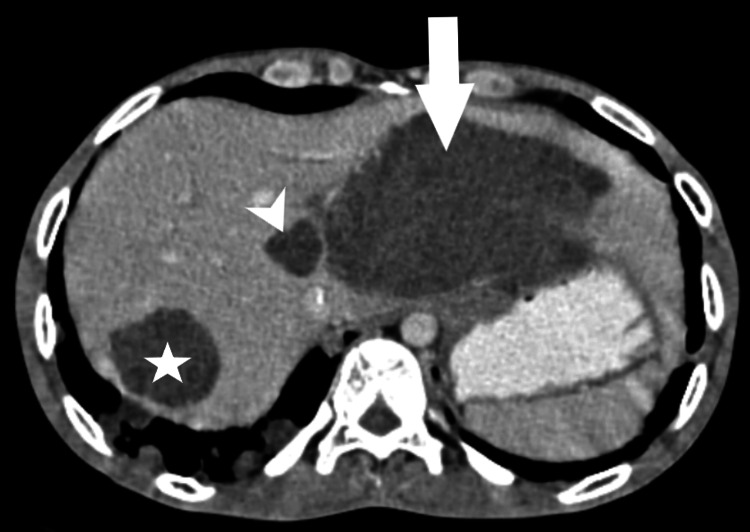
Contrast CT y axial abdomen venous phase showing multiple enhancing lesions in the liver (star and arrowhead), largest in segment VII (asterisk) CT: computed tomography
